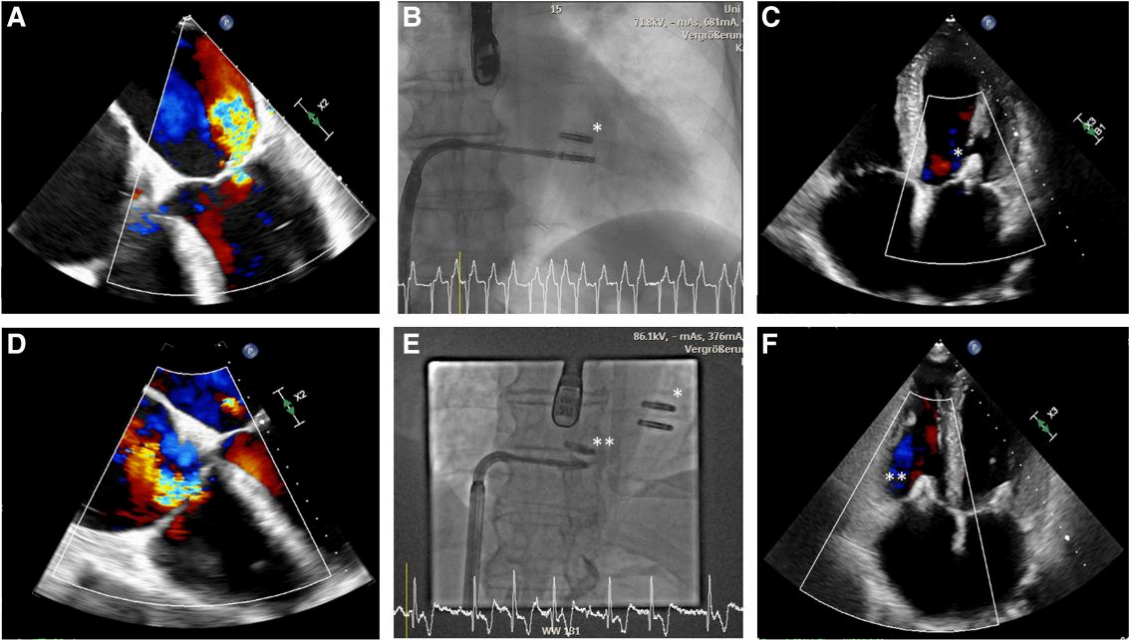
(*A*) TOE colour Doppler of MR before MV TEER. (*B*) TOE colour Doppler of TR before TV TEER. (*C*) AP fluoroscopic view as clips are inserted into MV. (*D*) TTE colour Doppler of MR after MV TEER. (*E*) AP fluoroscopic view as clips are inserted into TV. (*F*) TTE colour Doppler of TR after TV TEER. *Indicates PASCAL-Ace device in MV position. Indicates PASCAL-Ace device in TV position. TOE, transoesophageal echocardiogram; TEER, transcatheter edge-to-edge repair; TTE, transthoracic echocardiography; AP, anterior–posterior; MV, mitral valve; TV, tricuspid valve; MR, mitral regurgitation; TR, tricuspid regurgitation.
